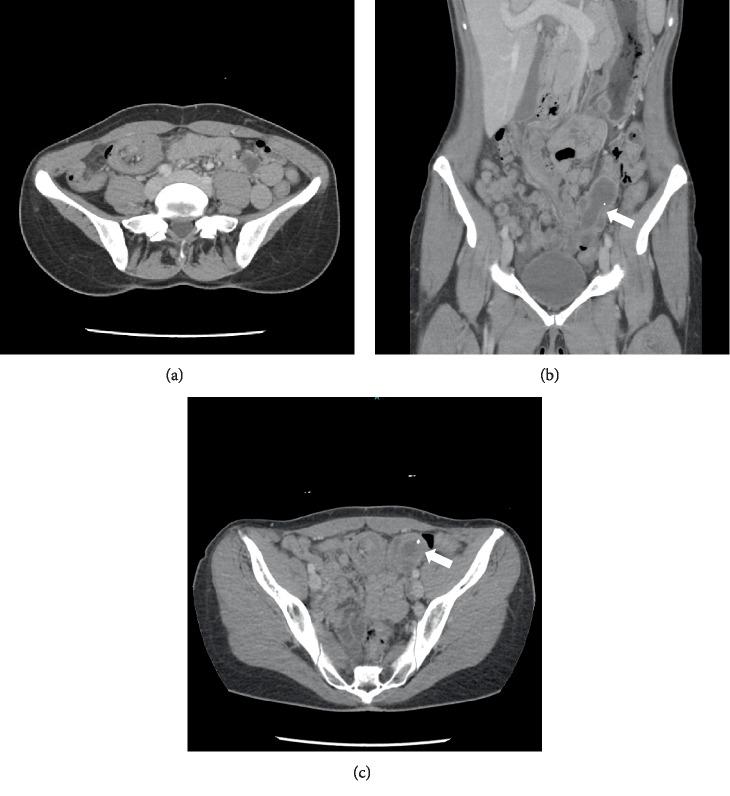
Axial and coronal CT images showing intussusception with the appendix indicated by the presence of a distended fluid-filled tube and an appendicolith (arrow).
